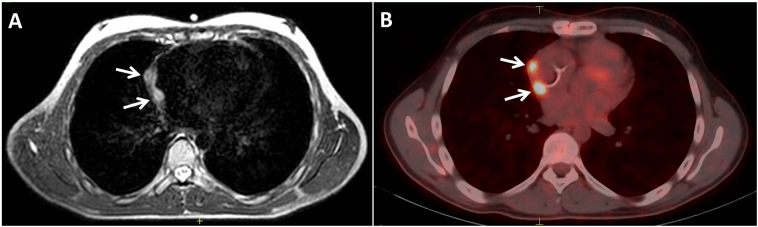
Recurrent nodular lesions (arrows) located at the right atrial wall demonstrated in (A) magnetic resonance imaging and (B) 18-flourodeoxyglucose positron emission tomography.
